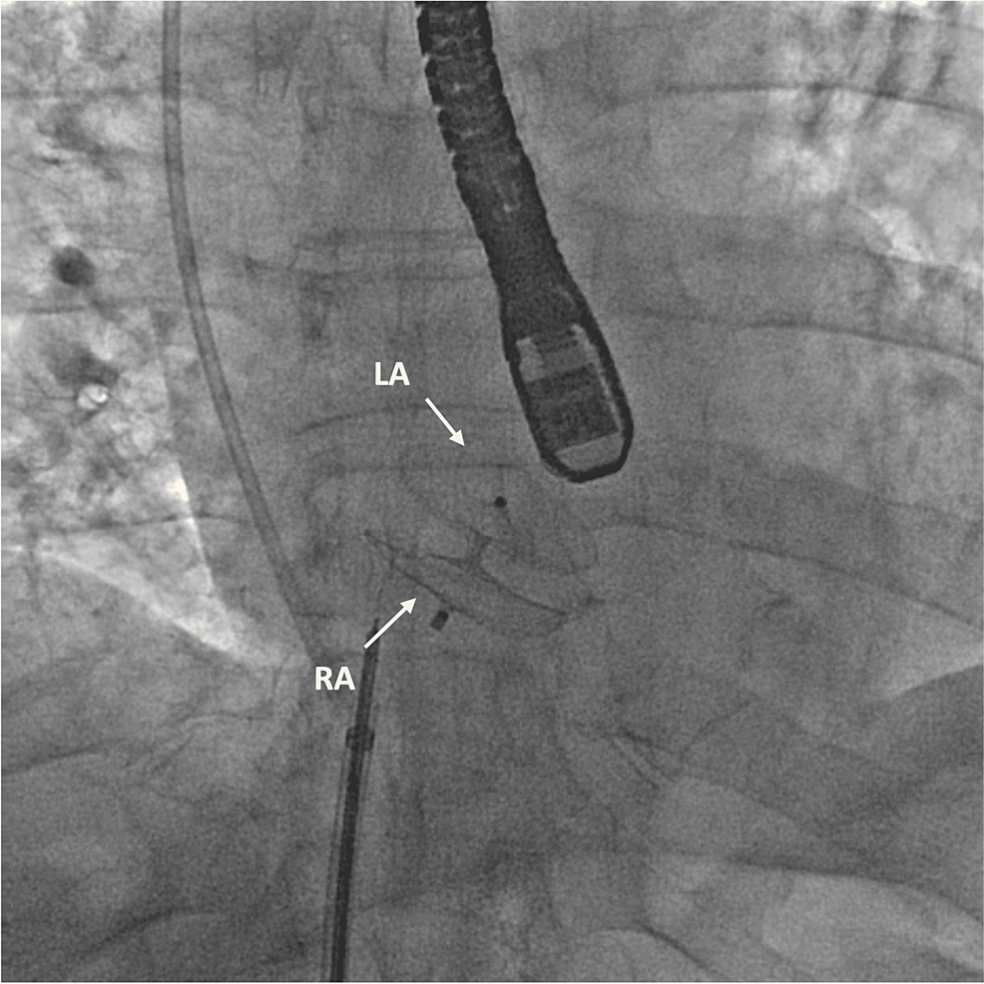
TEE- and fluoroscopy-guided PFO occluder device implantation Fluoroscopic image of the Abbott Amplatzer 30-mm PFO occluder device in interatrial septum with an anteroposterior view with arrows pointing to the left and right atrial disc deployed in the interatrial septum. TEE, transesophageal echocardiogram; PFO, patent foramen ovale; RA, right atrium; LA, left atrium
